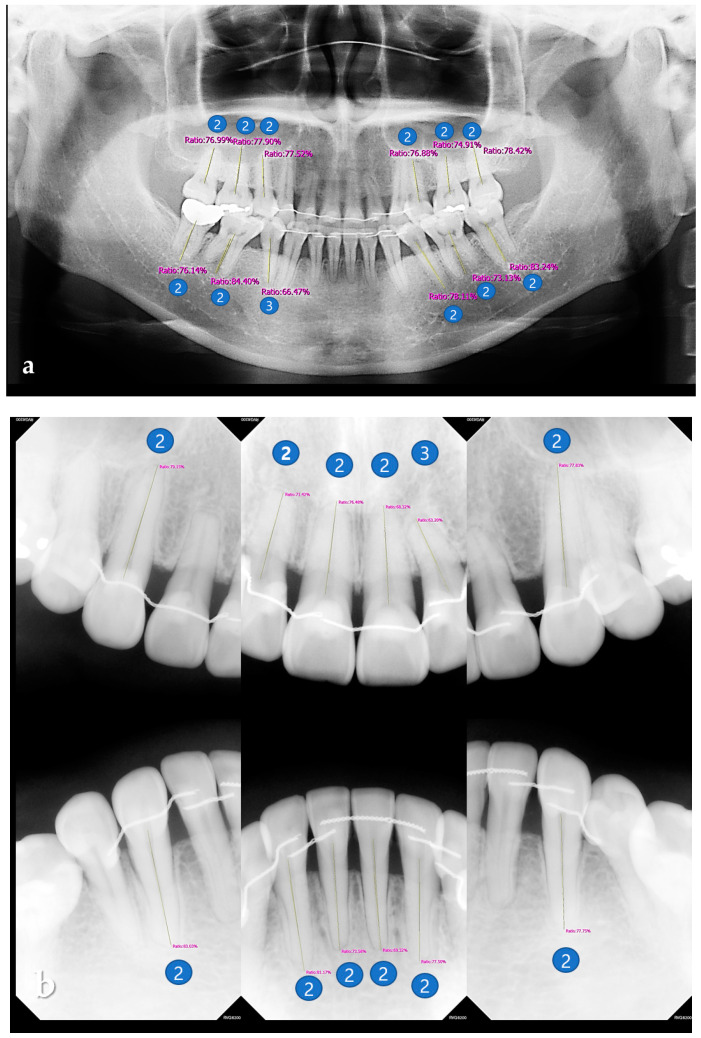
RBL scoring on a panoramic image (a) and periapical radiographic image (b) based on the percentage rate, to classify the periodontal bone loss of the tooth for periodontitis staging according to the new criteria. The classification criteria were as follows. Score 1: RBL < 15%. Score 2: 15% ≤ RBL ≤ 33%. Score 3: 34% ≤ RBL ≤ 66%. Score 4: RBL > 66%.
